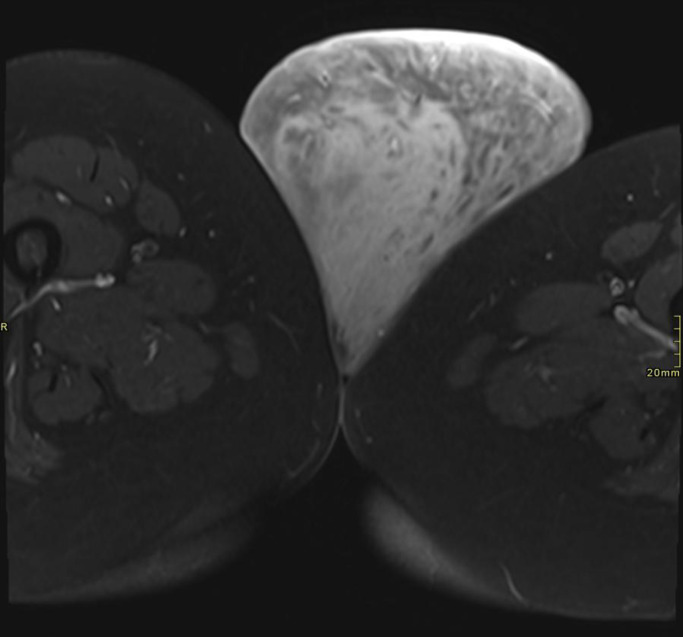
Axial T2-weighted MRI showing a large, well-defined, heterogeneously hyperintense mass arising from the left vulvar region, displacing adjacent soft tissues without invasion.
